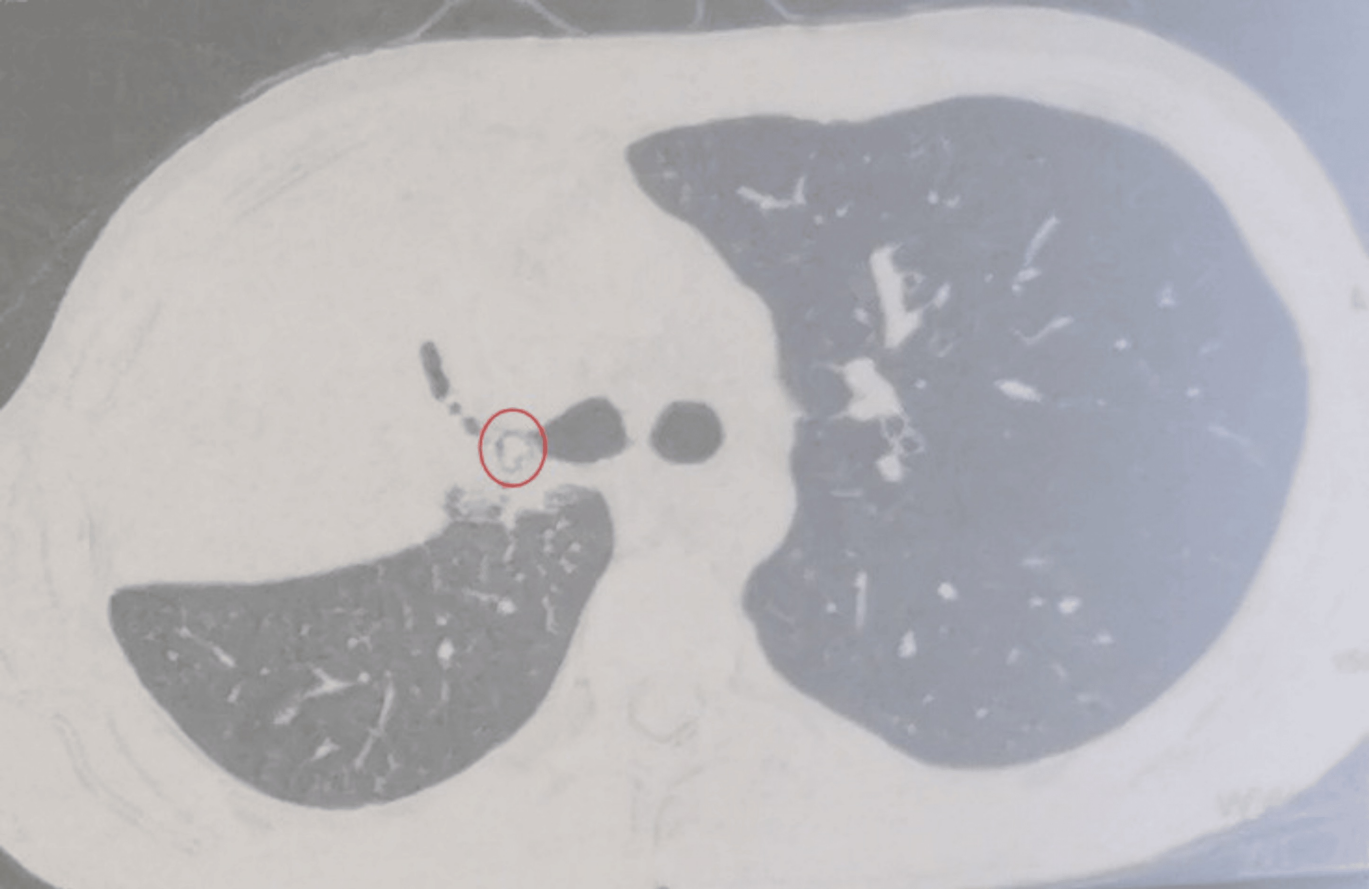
Axial CT scan showing a foreign body in the right upper lobe bronchus (red circle), associated with alveolar consolidation of the same lobe. CT, computed tomography
